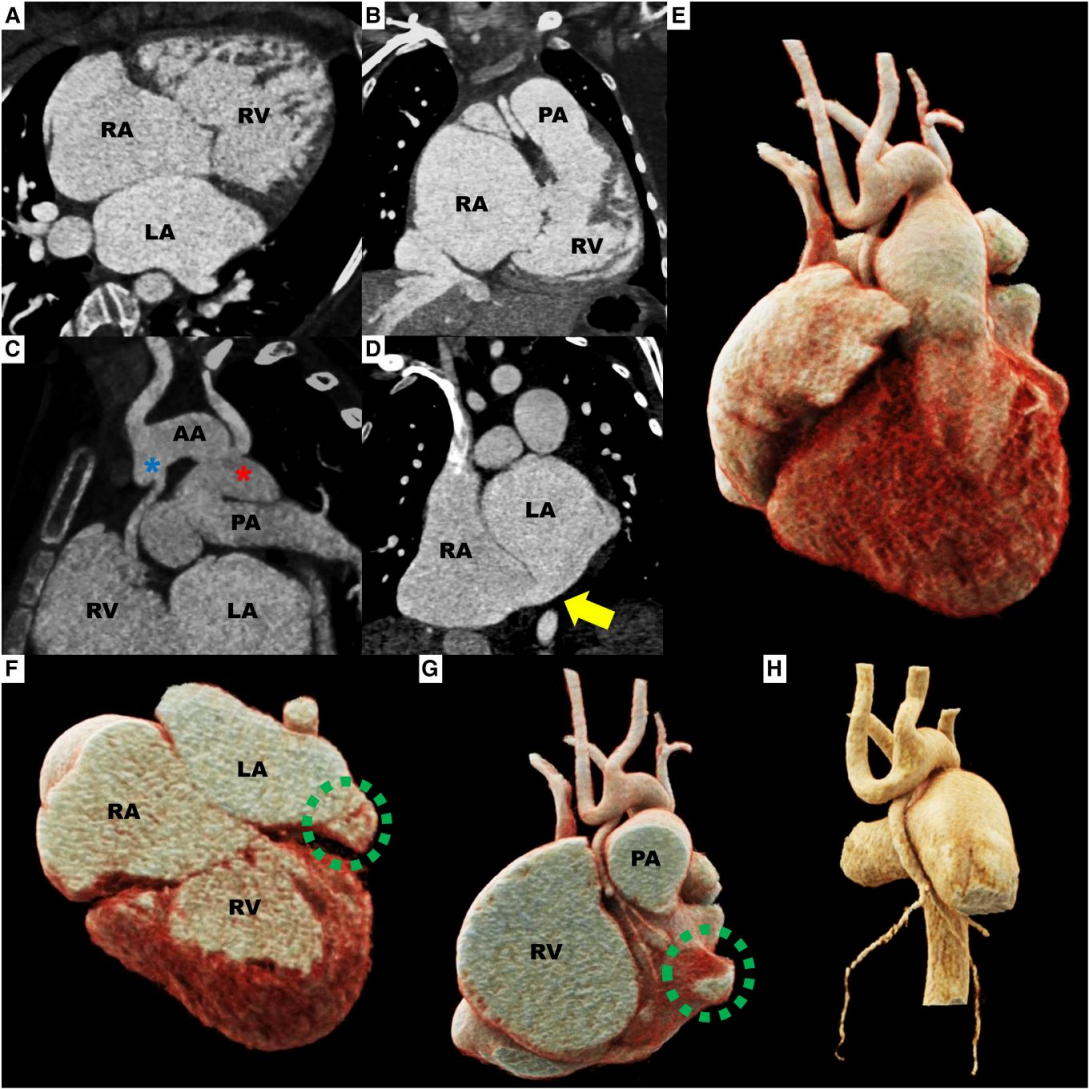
Cardiac computed tomography. Usual atrial arrangement, concordant atrioventricular connections with an imperforate left atrioventricular junction due to mitral atresia, a rudimentary morphologic left ventricle positioned posteriorly and to the left (green dotted line), and ventriculoarterial connections of the single-outlet type from the morphologic right ventricle secondary to AA, sinus venosus atrial septal defect measuring 9 mm × 12 mm (yellow arrow), coronary arteries originating bilaterally from a hypoplastic ascending aorta (blue asterisk), and a prominent patent ductus arteriosus measuring 17 mm × 20 mm at the pulmonary end, 18 mm × 18 mm at the aortic end, and 5 mm in length (red asterisk). (*A*) Axial MPR view; (*B*) coronal MPR view; (*C*) oblique MIP view; (*D*) coronal MPR view; (*E*) cinematic VRT 3D reconstruction in anterior view; (*F*) slice-based cinematic VRT 3D reconstruction in axial view; (*G*) slice-based cinematic VRT 3D reconstruction in coronal view; (*H*) cinematic VRT 3D reconstruction of coronary anatomy. 3D, three-dimensional; AA, ascending aorta; LA, left atrium; MIP, maximum intensity projection; MPR, multiplanar reconstruction; PA, pulmonary artery; RA, right atrium; RV, right ventricle; VRT, volume rendering technique.
